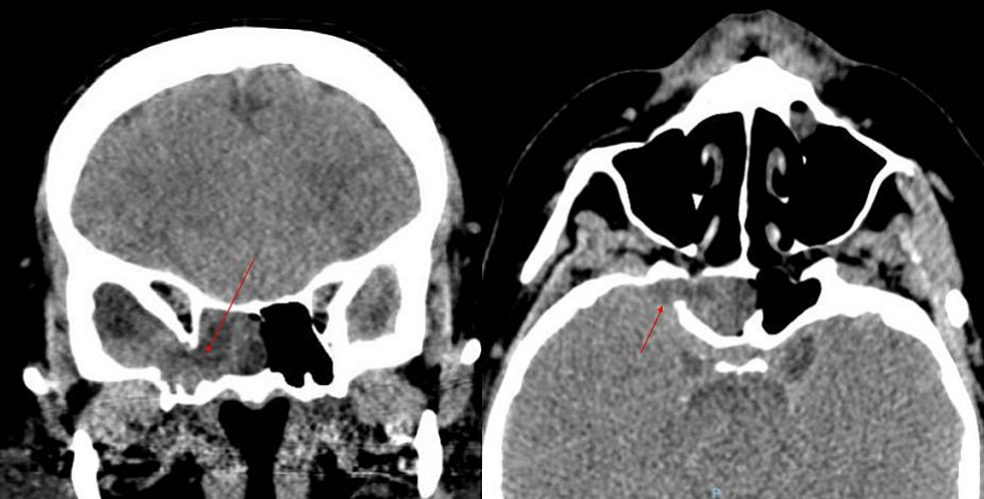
Head computed tomographic scan shows a 1.1 cm osseous defect at the lateral wall of a right sphenoid sinus (red arrow), resulting in direct communication between the middle cranial fossa and the right sphenoid sinus Coronal (left), axial (right)
